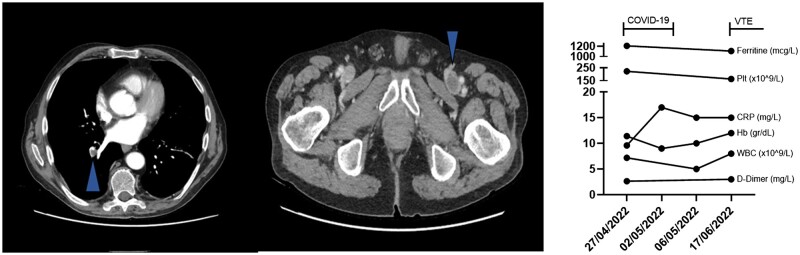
CT scan of the patient and time course of laboratory parameters. Arrowheads indicate thrombotic material in the left femoral vein and in the segmental posterior basal branch of the right pulmonary artery. Hb: Haemoglobin; Plt: Platelets; VTE: venous thromboembolism; WBC: white blood cells
